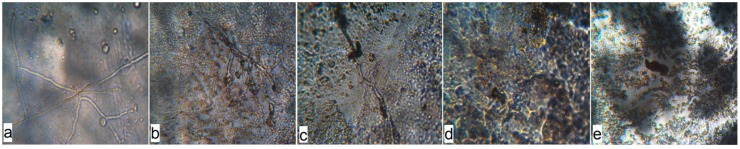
Microphotographs of the surface of the inoculated polyethylene materials with birch bark extract and with the use of ultrasonic treatment on the 14th day of *A. niger* exposure (100× magnification): (a) PE 0% BBE ultrasound; (b) PE 0.5% BBE, ultrasound; (c) PE 1.0% BBE, ultrasound; (d) PE 2.0% BBE, ultrasound; (e) PE 5.0% BBE, ultrasound.
